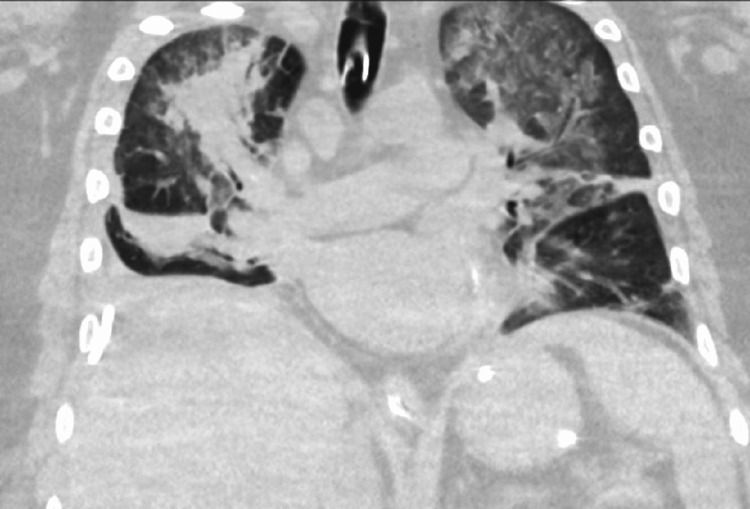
Interval CT chest demonstrating worsening of pulmonary infiltrates
